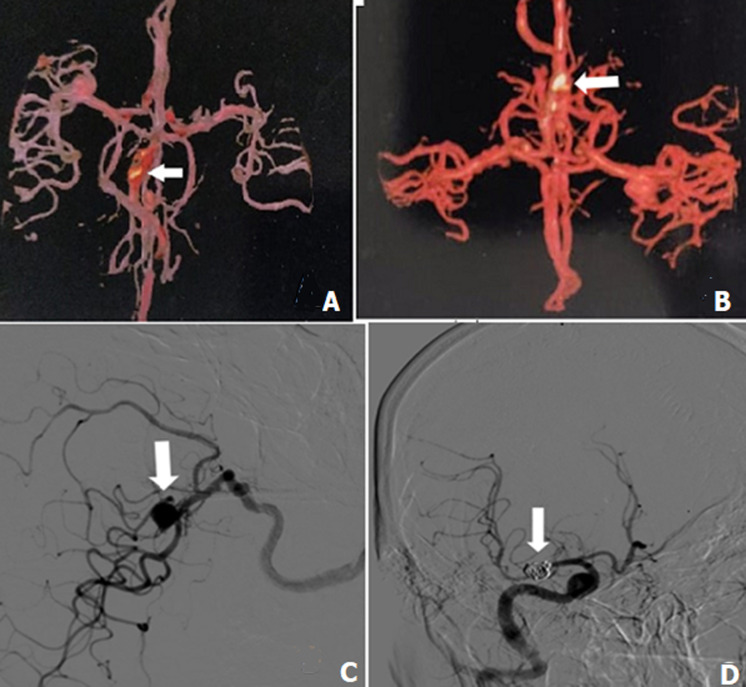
showing angiography reports pre and post aneurysm coiling and embolization; A,B) pre-operative multi-slice CT angiography of Brain arteries revealing Aneurysm in right middle cerebral artery; C) digital subtraction angiography (intravenous) pre-operative, showing right middle cerebral artery aneurysm (arrows); D) post-operative digital intravenous angiogram showing (arrow) coiling of the aneurysm and embolization visible through restricted blood flow to the coiled area
